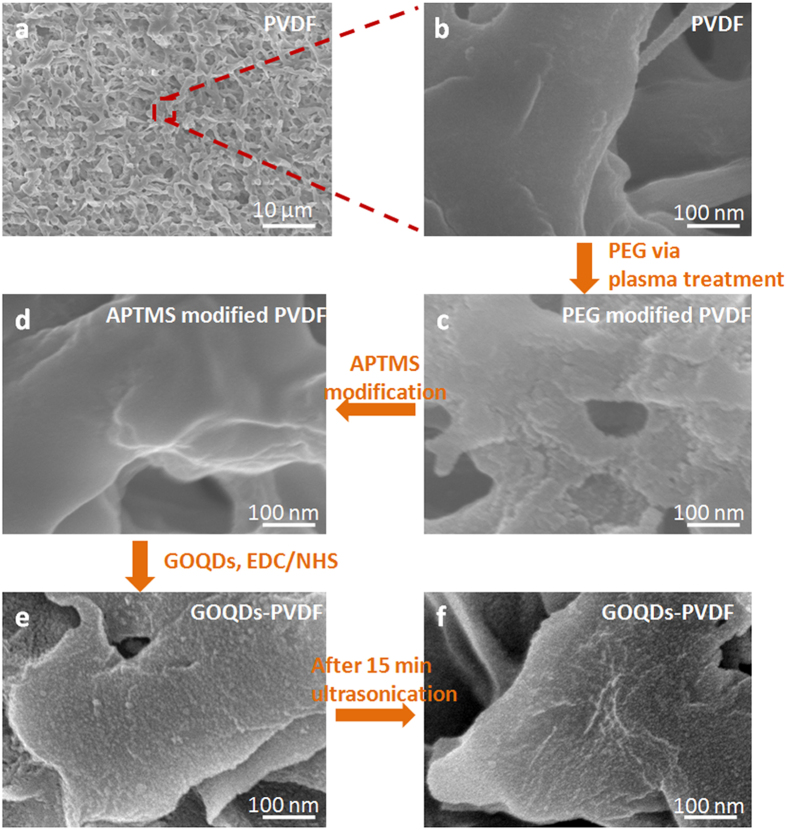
SEM images of (a,b) pristine PVDF, (c) PEG modified PVDF, (d) APTMS modified PVDF, (e) GOQDs-PVDF and (f) GOQDs-PVDF membranes after 15 min ultrasonication treatment.
